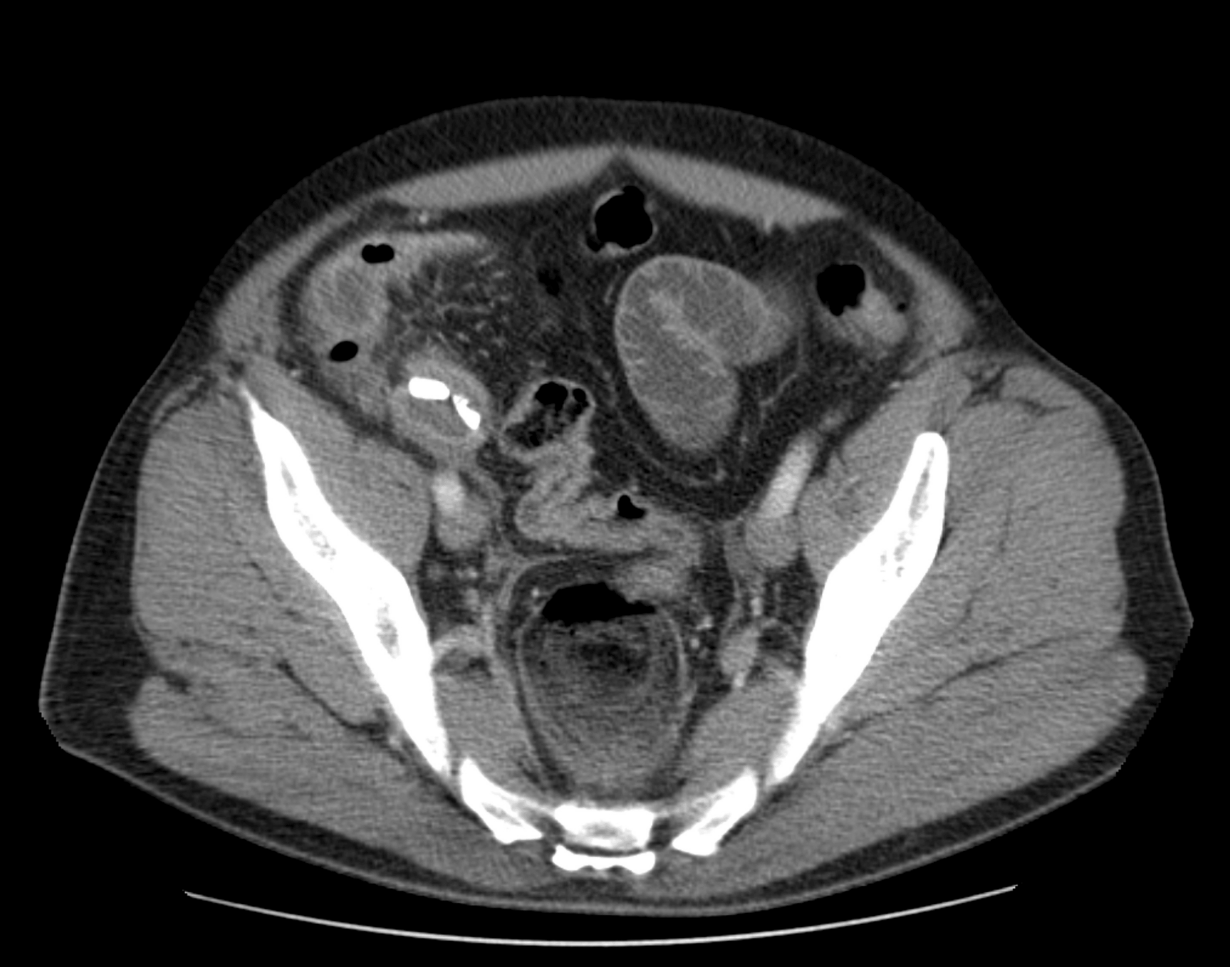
Transverse view of CT scan with the foreign body pictured
